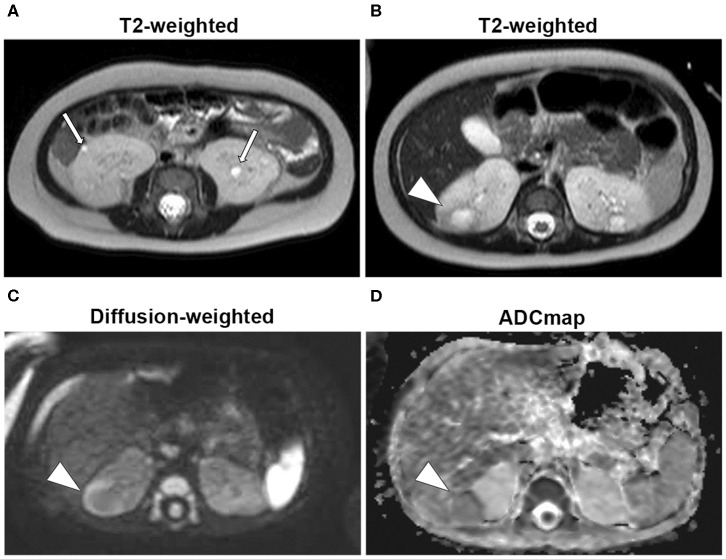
Magnetic resonance imaging (Day 60). (A) T2-weighted images: 2-mm high-signal small patchy shadows were found in both kidneys (arrow). (B) T2-weighted images: thick walls in the right kidney as low signals and mass as high signals were found in the interior (arrowhead). (C) Diffusion-weighted images: masses as high-signal areas of thick-walled structures at the same site as (B) (arrowhead). (D) Apparent diffusion coefficient (ADC) map: low-signal mass at the same site as (B) (arrowhead).
